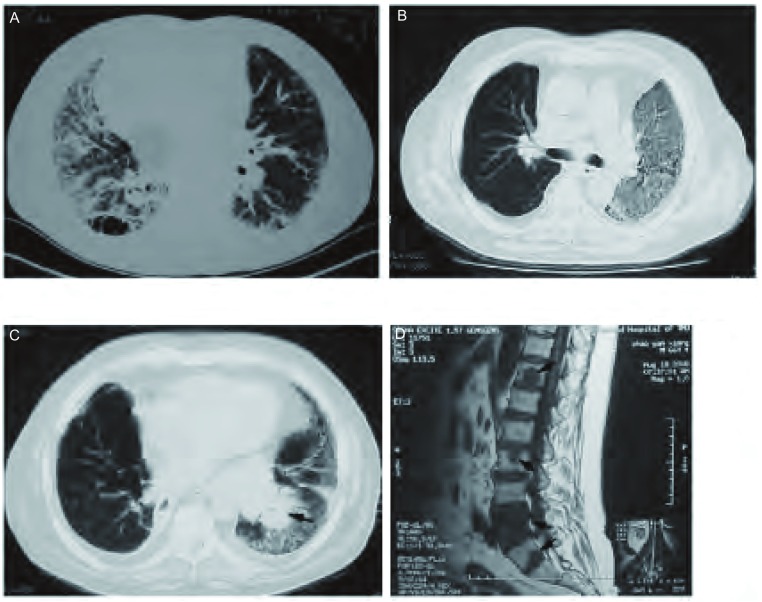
患者临床影像学特征。A：术前CT扫描显示两肺弥漫性纤维化，呈蜂窝样改变，尤其以右肺明显；B：术后一月复查CT显示移植肺扩张良好，左肺弥漫性纤维化加重；C：肺移植术后13个月复查CT显示左肺弥漫性纤维化，左下肺心缘团块影；D：同期行MRI，显示有多发骨转移（T12、L3、L5、S1）。 Clinical radiologic features of the patient. A: Preoperative HRCT showing predominant reticular abnormality with honeycombing, particularly in the right lung; B: Postoperative HRCT (1 month) showing satisfactory reexpansion of the transplanted lung and progression of lung fibrosis in the left native lung; C: Postoperative HRCT (13 months) showing fibrosis in the left lung and a pulmonary mass in the left lower lobe (cardiac border, arrow); D: Postoperative MRI (13 months) showing multiple osseous metastases in the vertebral column (T12、L3、L5、S1, arrows).
